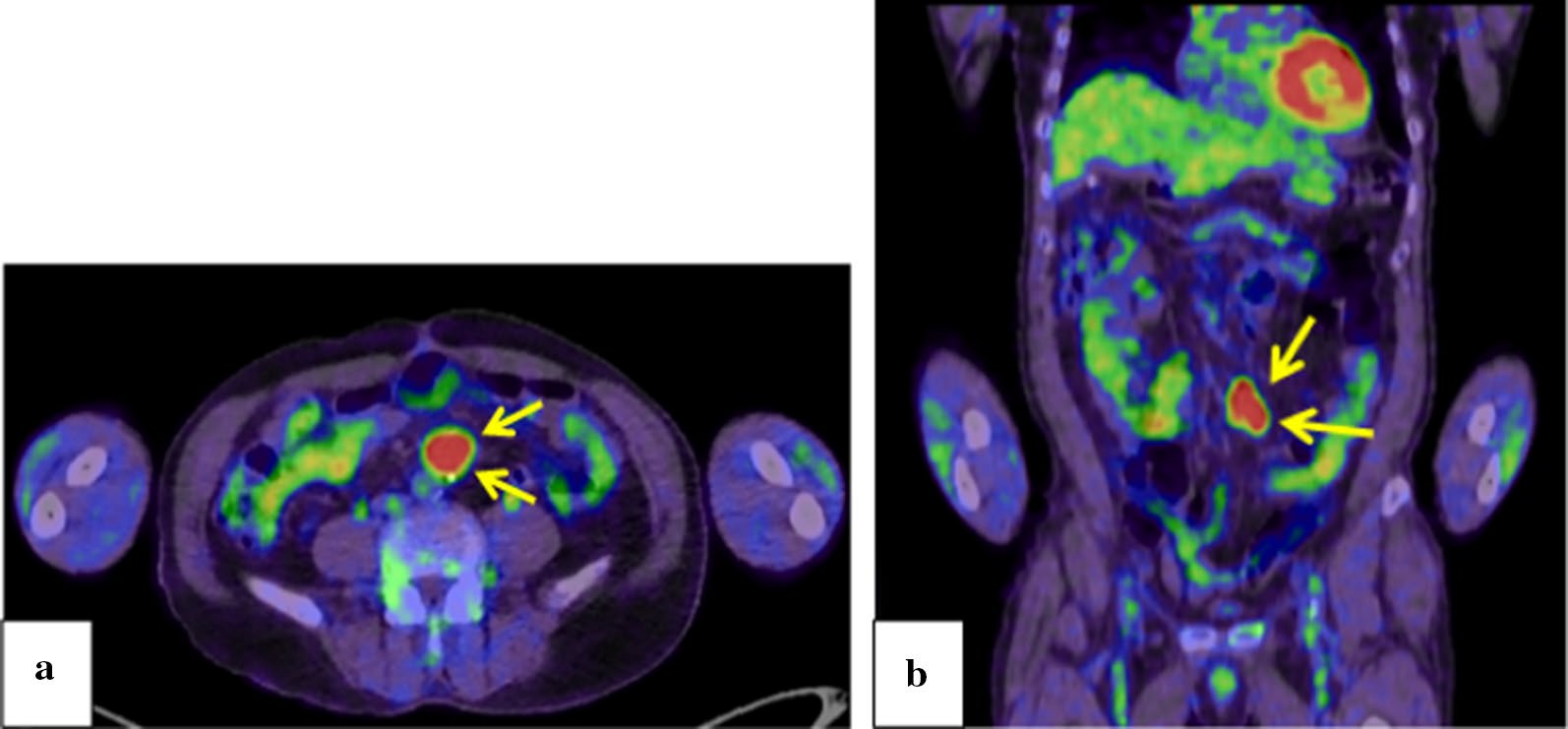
a, b PET revealed FDG accumulated only into the para-aortic lymph node
